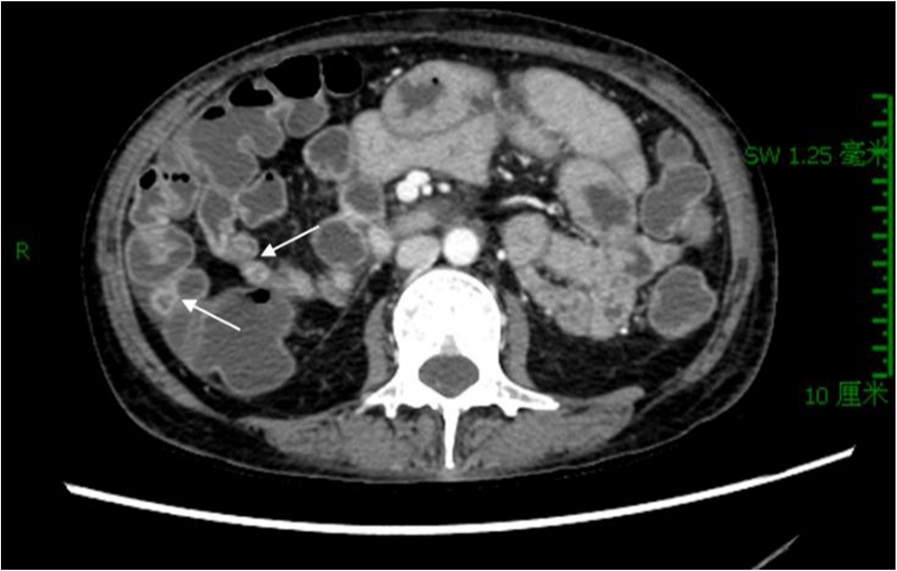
Radiograph of the abdominal computed tomography (CT). The findings show segmental bowel walls are thickened (arrows)
